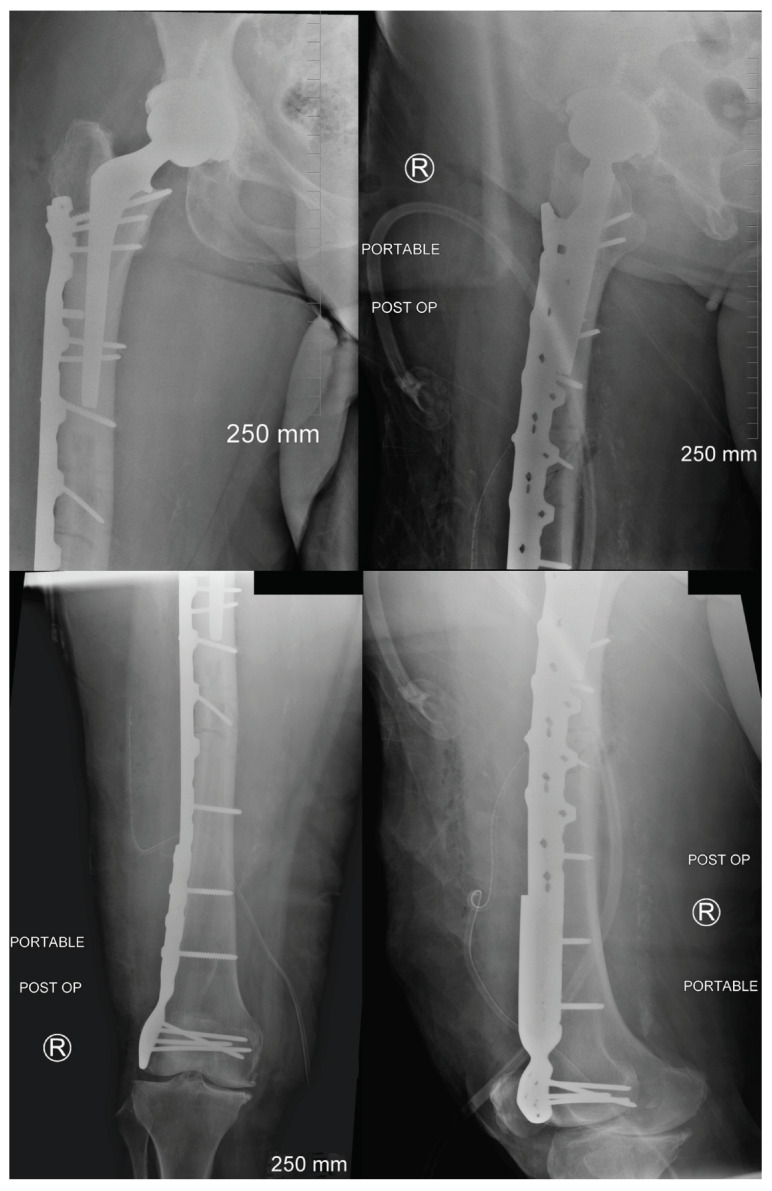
Immediate postoperative radiographs following open reduction and internal fixation with a lateral periprosthetic femoral plate.
